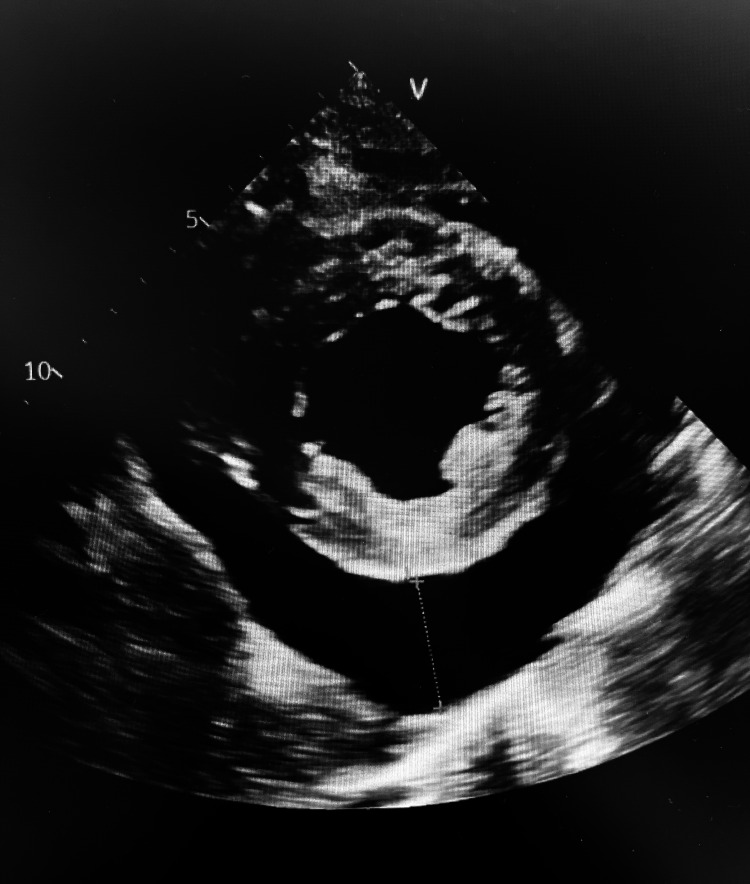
Transthoracic echocardiogram short-axis view showing a large pericardial effusion.
